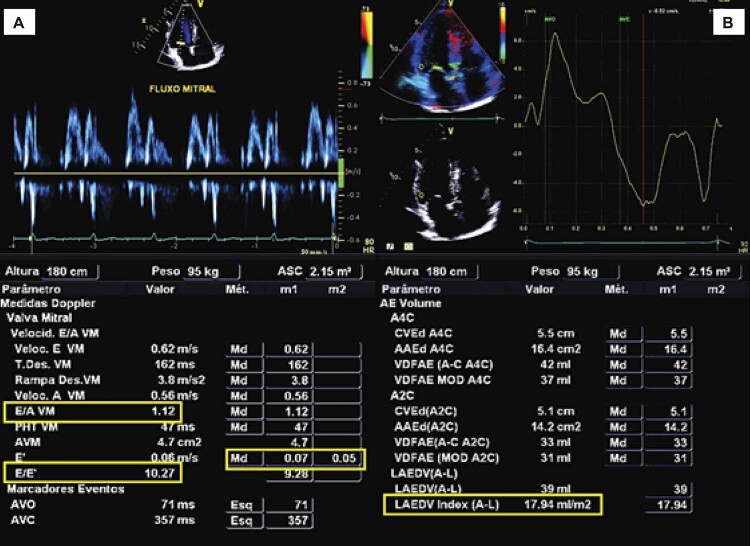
Curva do fluxo mitral ao Doppler pulsátil (superior esquerda); curva do Doppler tecidual do anel mitral lateral (superior direita). E/A VM: relação E/A da valva mitral; LAEDVindex(A-L): volume indexado do átrio esquerdo.*
